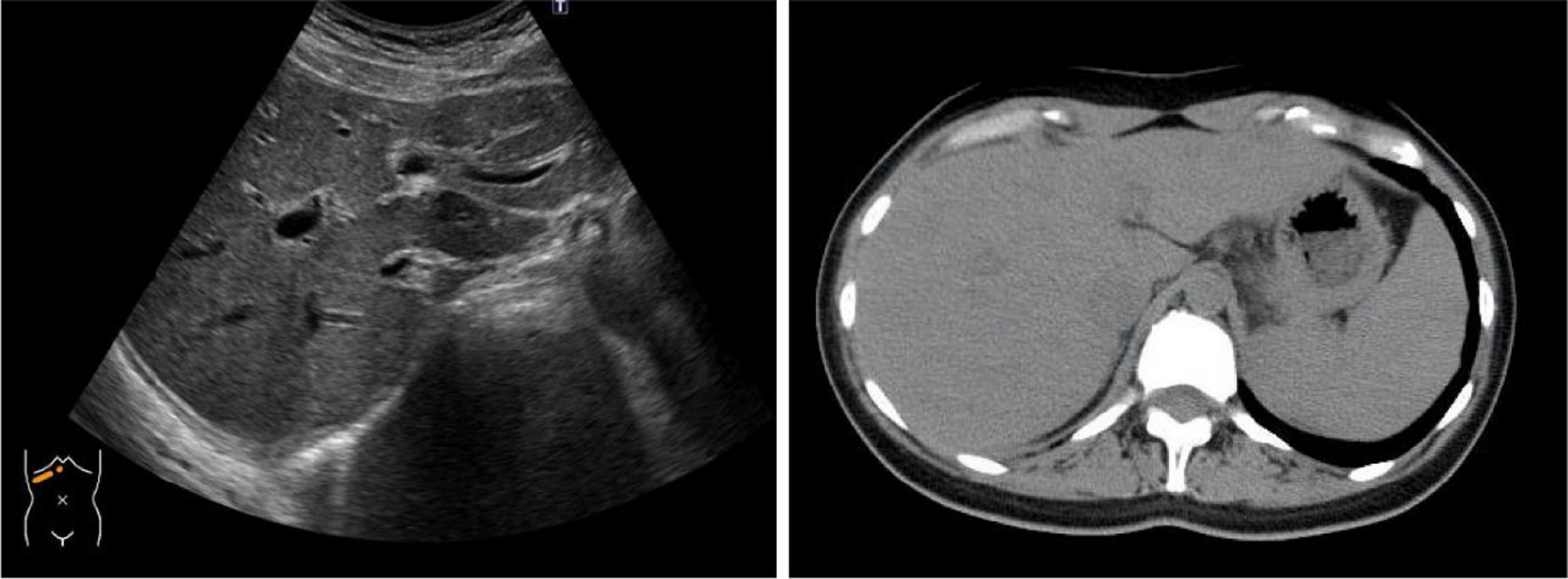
Abdominal ultrasound and computed tomography scans revealed mild hepatosplenomegaly and periportal edema. The echo levels of the liver are decreased and crude.
